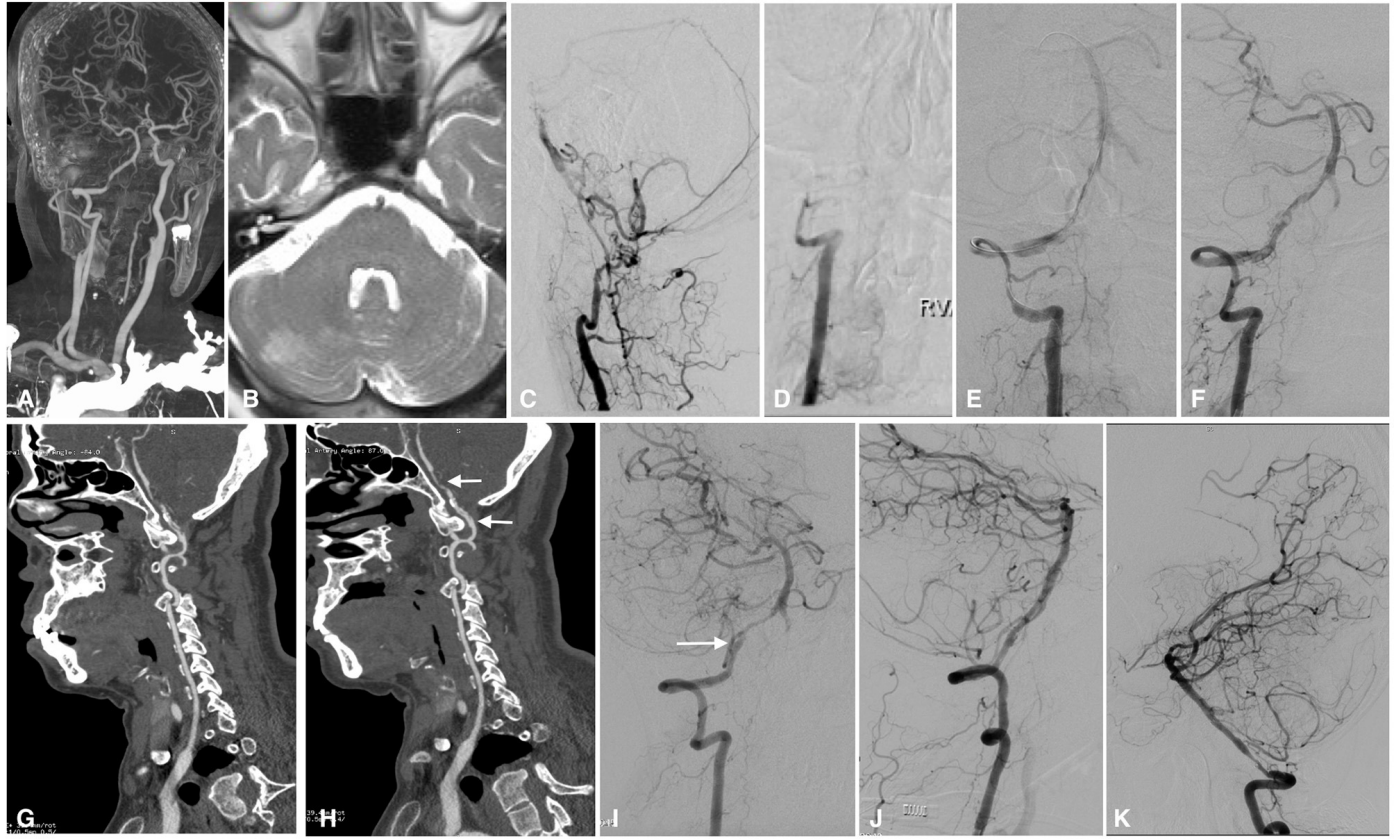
Imaging studies of case 3. CTA in a local hospital showed severe stenosis of the right intracranial vertebral artery 6 months before admission (A). Axial T2-weighted MRI showed ischemic infarction in the right cerebellum (B). The left intracranial vertebral artery was occluded, and the BA was supplied by the anastomotic branches and leptomeningeal collaterals (C). The right intracranial vertebral artery was occluded from the V3 segment onward (D). In the first stage of endovascular treatment, the right intracranial vertebral artery was recanalized by balloon inflation (E), and stable perfusion was achieved after sufficient dilation with 3 balloons; however, some thrombus remained on the vessel wall (F). CTA performed 1 week later showed intraluminal thrombus and a dissection in the right intracranial vertebral artery (G). Two weeks later, CTA showed that the thrombus had decreased significantly, leaving a dissection and residual stenosis (H). Angiography performed in the second stage confirmed that the thrombus had disappeared and that there was a dissection (white arrow) and residual stenosis in the V4 segment of the intracranial vertebral artery (I); the dissection and stenosis were resolved by a Winspan stent (J). Follow-up angiography performed 12 months later showed moderate stenosis in the proximal part of the stent (K). CTA, computed tomography angiography; MRI, magnetic resonance imaging; BA, basilar artery.
